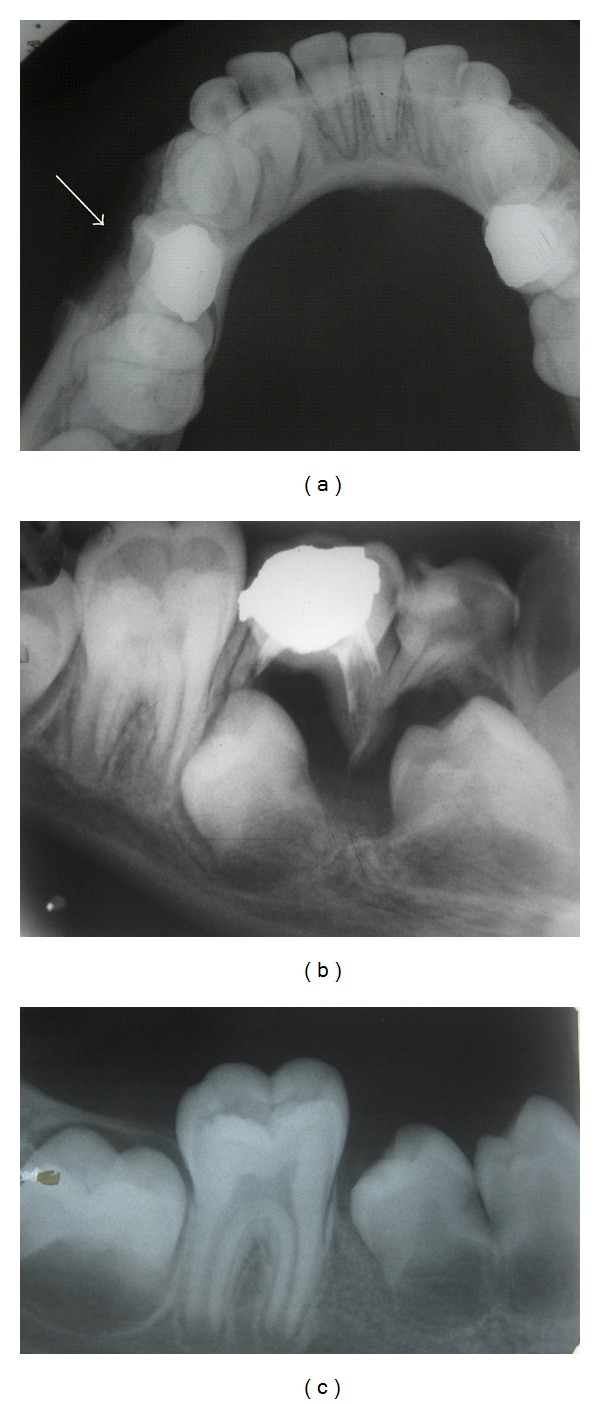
(a) Mandibular occlusal radiograph in case 2 showing erosion of the buccal cortex of the mandible. (b) Intraoral periapical radiograph showing radiolucency in relation to 84, 85 along with displacement of the underlying successor teeth. (c) Intraoral periapical radiograph showing eruption of 44, 45 following extraction of 84, 85 and periapical curettage.
